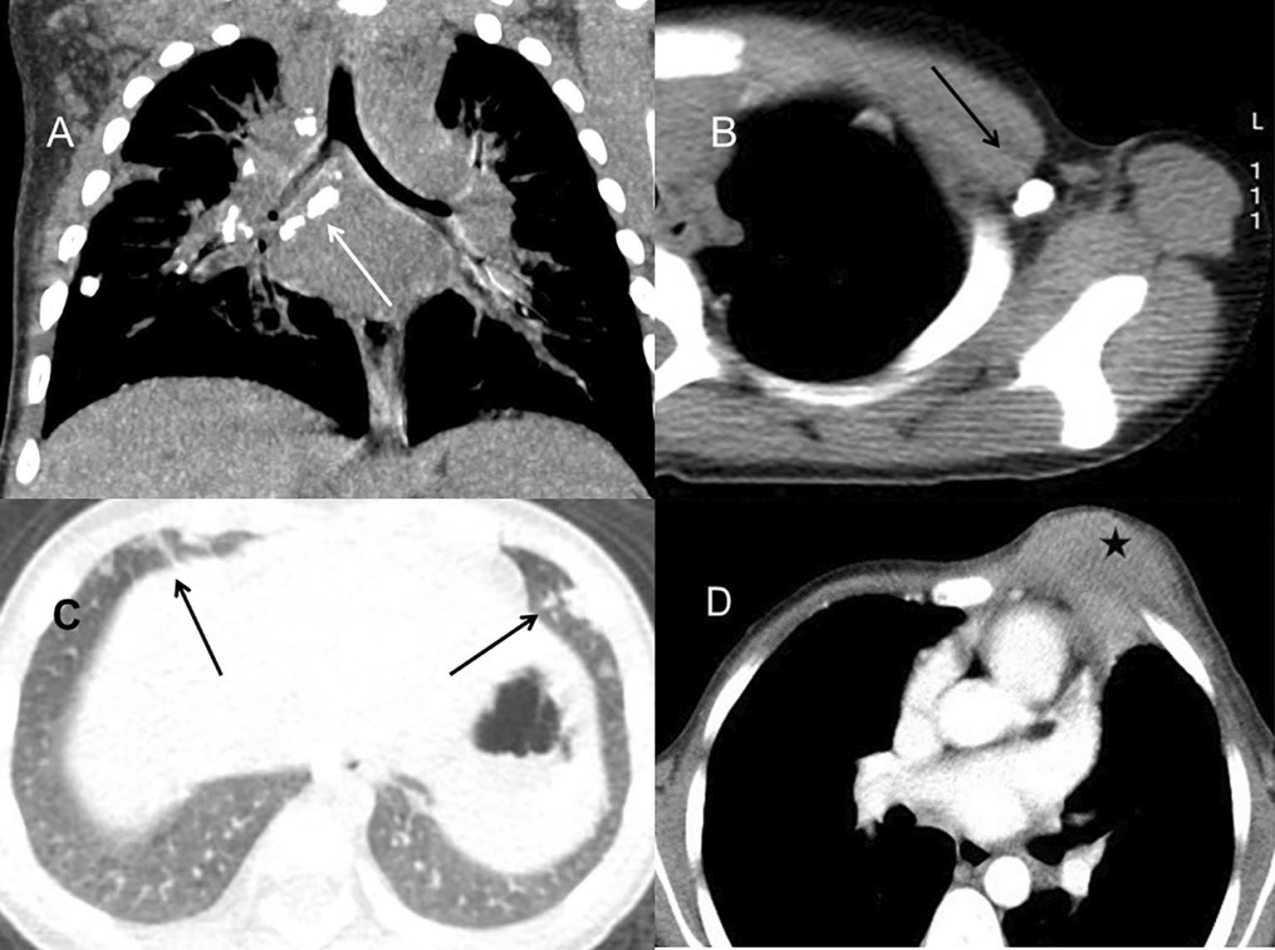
Pulmonary CT of BCG infection. (A) Coronal reformatted CT image of a 1-year-old boy demonstrated multiple nodular calcification within the mediastinal and hilar lymph nodes (long arrow). The calcified nodule in the right lower lobe was also found. (B) The left axillary calcification ipsilateral to the BCG injection site was found in a 1-year-old boy (long arrow). (C) Chest CT of a 9-month-old boy showed multiple pulmonary nodules in the bilateral lower lobe beneath the pleura (long arrow). (D) A 8-month-old boy with chest wall invasion. CT demonstrated a large chest wall mass with patchy areas of low density inside, suggesting the forming of abscess (star).
